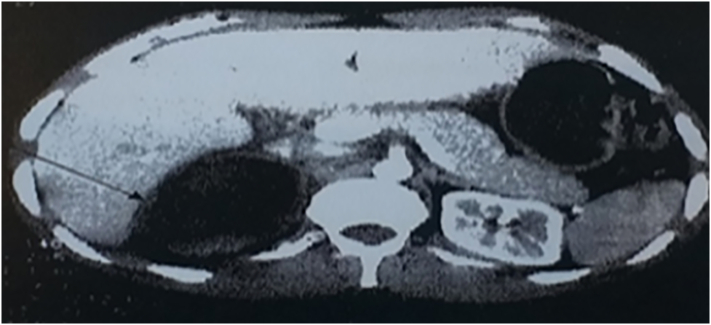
abdominal CT scan which revealed a 6/7 cm right adrenal mass of tissue density and cystic fibrosis.
